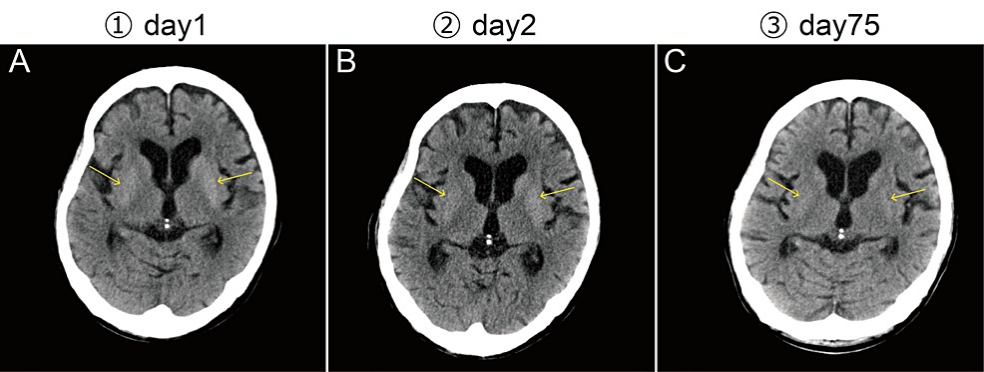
CT images CT showed hyperdensity in the bilateral putamen (arrow). On day 75, hyperdensity in the bilateral putamen was attenuated compared with that on days 1 and 2. The number of days was counted from the date of first admission.
